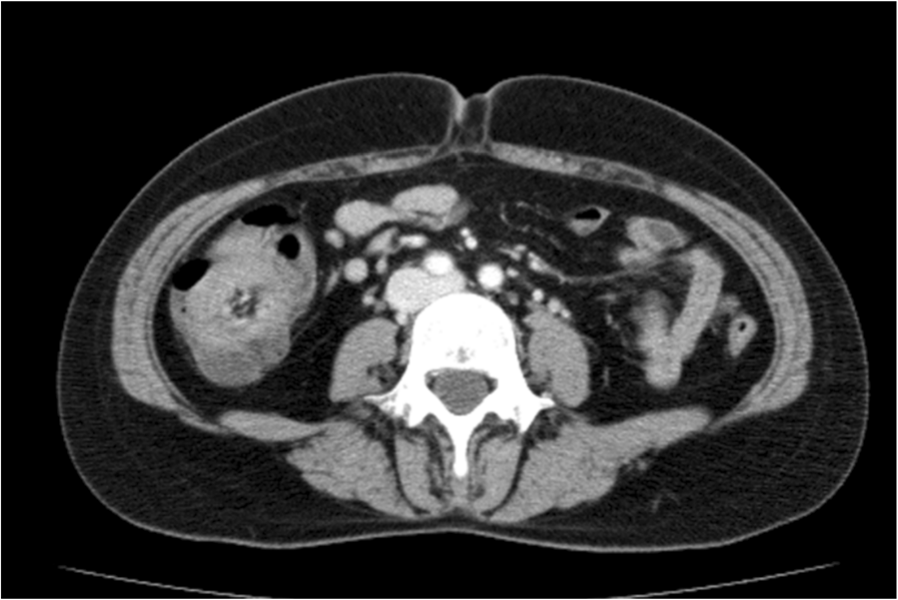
Abdominal computed tomography showing cecal wall thickening
